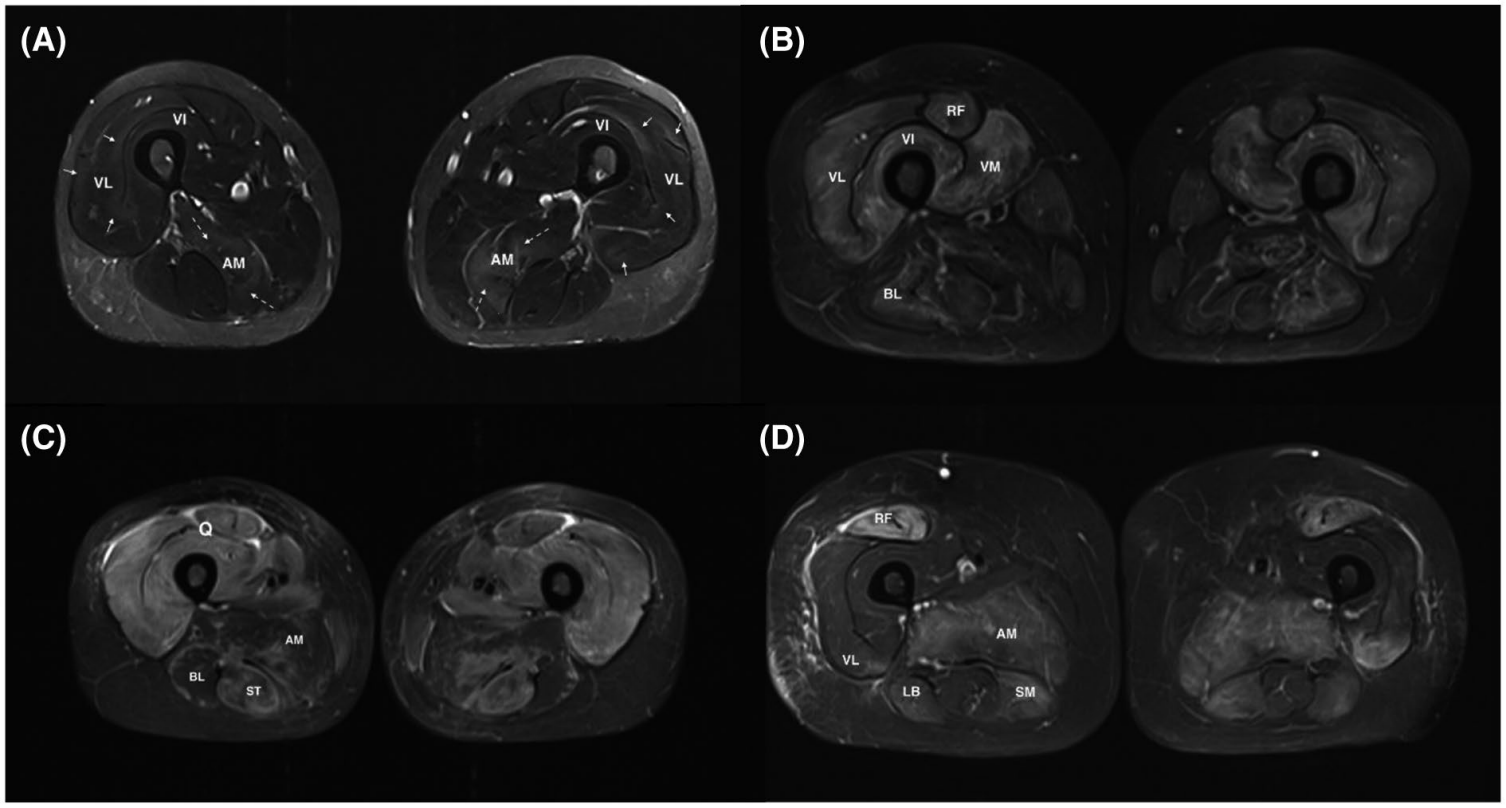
High muscle T2 signals in myositis: (A) Muscle MRI of an overlap myositis (scleroderma) showing mild muscular inflammation. Normal muscles appear with a very low signal (close to the subcutaneous tissue after fat signal suppression). Inflammatory muscles exhibit high signal (slight) with blurred borders, clustered along the aponeuroses and muscular septa (full arrows). The dashed arrows show an area with a more intense hypersignal. (B) Myositis with moderate to severe muscle inflammation. MRI of a dermatomyositis patient showing hyperintense areas affecting mainly the four heads of the quadriceps muscle. (C) ASyS patient with severe muscle inflammation, displaying a marked T2 hyperintensity affecting all three compartments of the thighs on both sides. (D) MRI of an IMNM patient showing that hypersignal is also present when muscle fiber necrosis occurs in absence of significant inflammatory cell infiltratation. All pictures show thigh muscles MRI images (axial plane, T2 STIR w. seq). AM, Adductor magnus; LB, long biceps femoris; Q, quadriceps muscle; RF, rectus femoris; ST, semi‐tendinosus hamstring muscle; VI, vastus intermedius; VL, vastus lateralis; VM, vastus intermedialis
